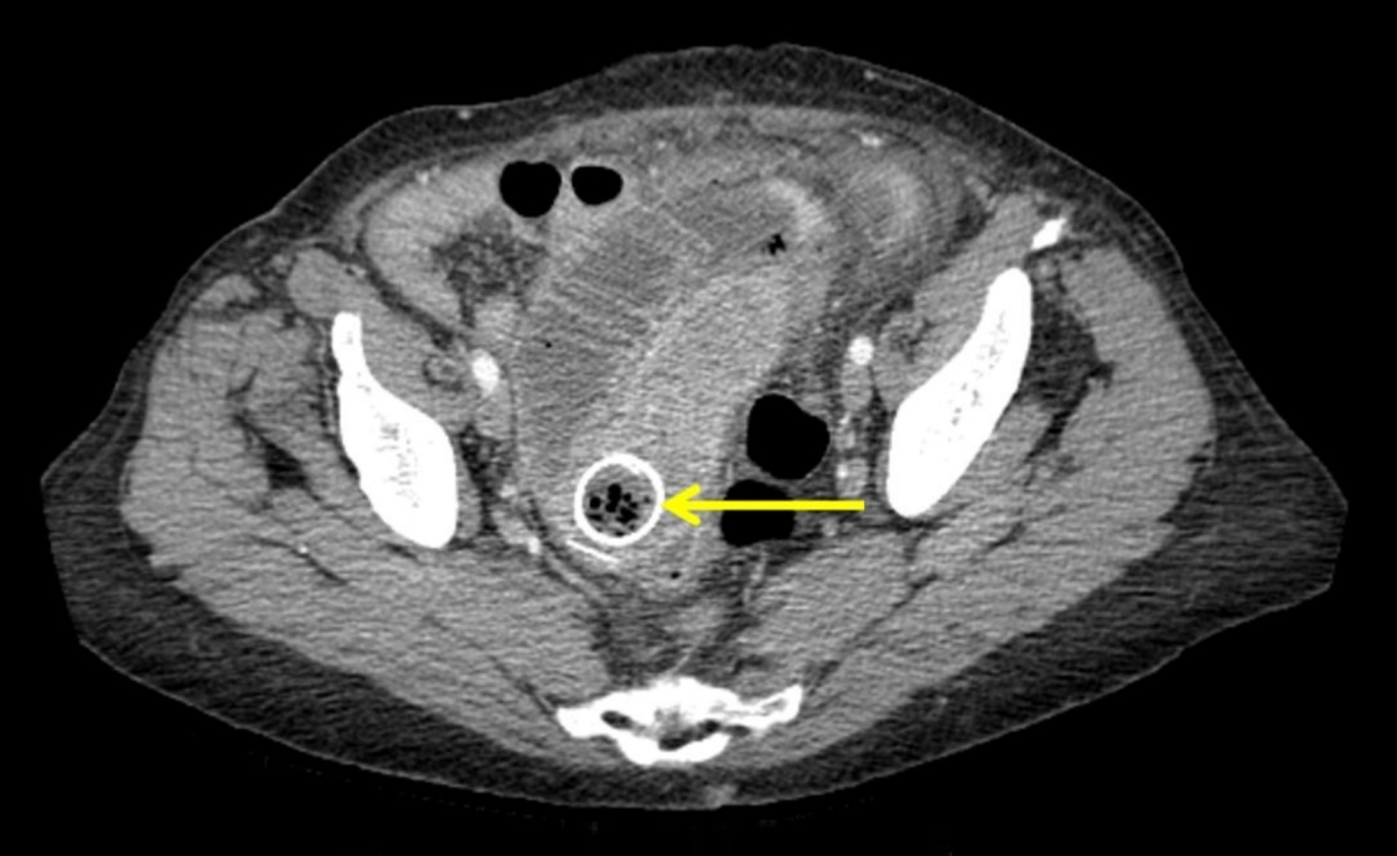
Computed tomography abdomen showing migrated stent located in the left-lower quadrant of the abdomen causing obstruction with the presence of free air in the abdominal cavity (Axial view). Arrow indicates the precise location of the stent.
